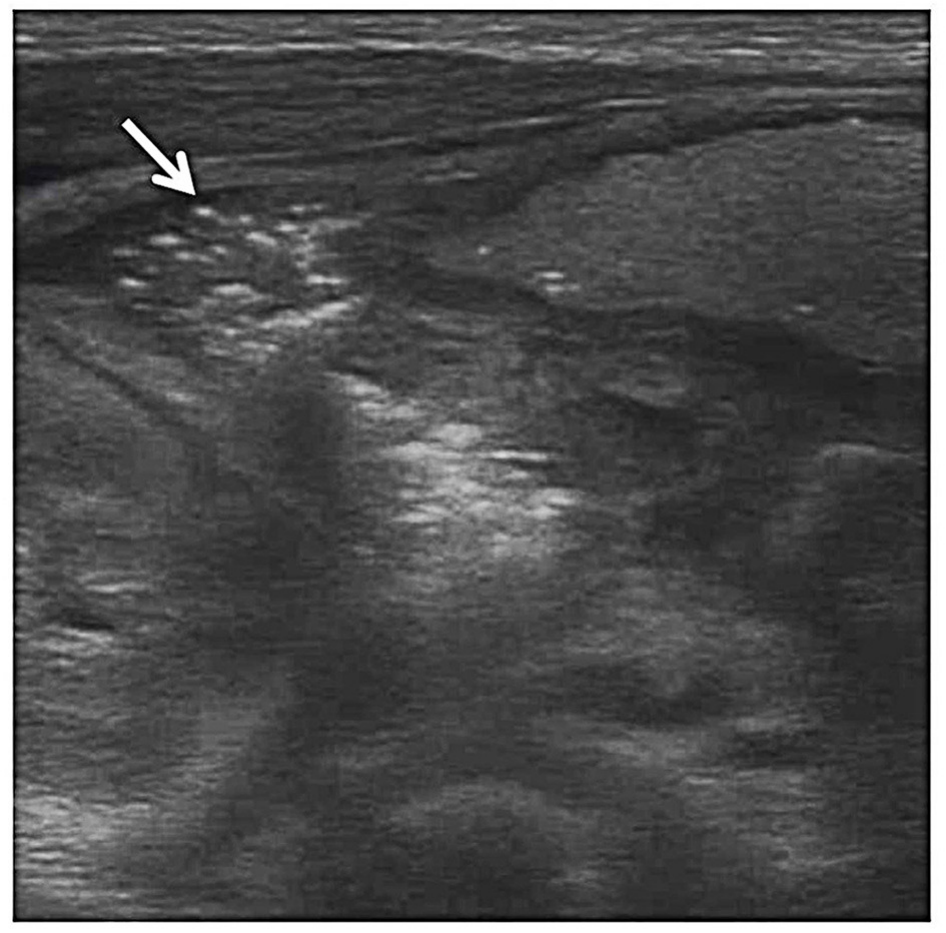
Pneumatosis intestinalis. Grayscale ultrasound shows echogenic foci (arrow) within the bowel wall indicative of gas bubbles within the bowel wall.
